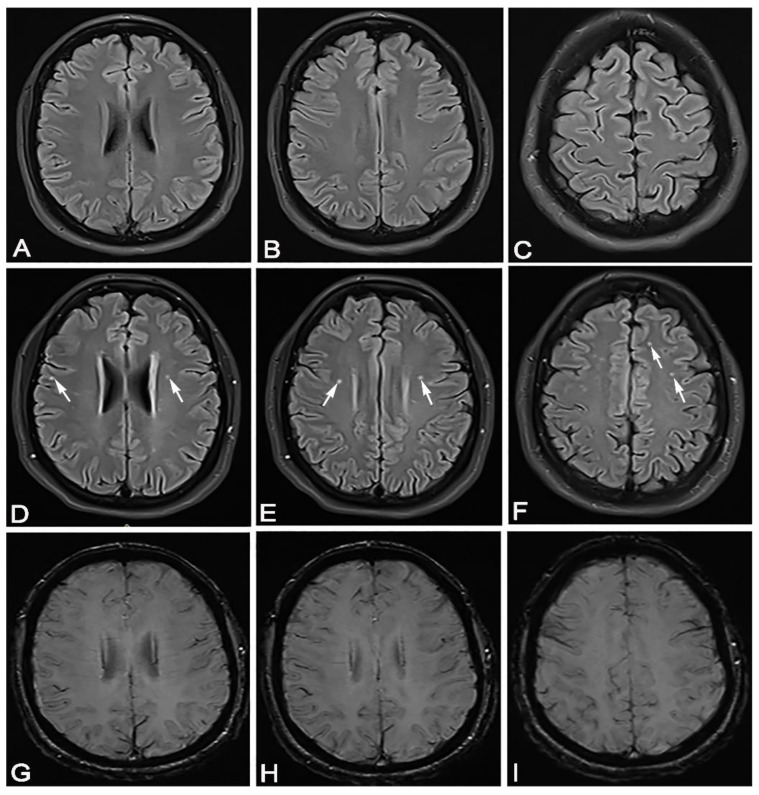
In April 2020, a 35-year-old female patient came for a brain MRI due to vertigo. (A–C) She showed completely normal brain MRI. In February 2021, 50 days after recovering from mild SARS-CoV-2 infection, the same patient came for a brain MRI due to headache, vertigo and taste and smell disorder. (D–F) She showed hyperintense lesions on FLAIR sequence, periventricular bilaterally, bifrontal subcortical and right juxtacortical (white arrows). The same transverse cross sections of the FLAIR sequence as on (A–C), without signs of microhemorrhage on the SWI sequence (G–I).
